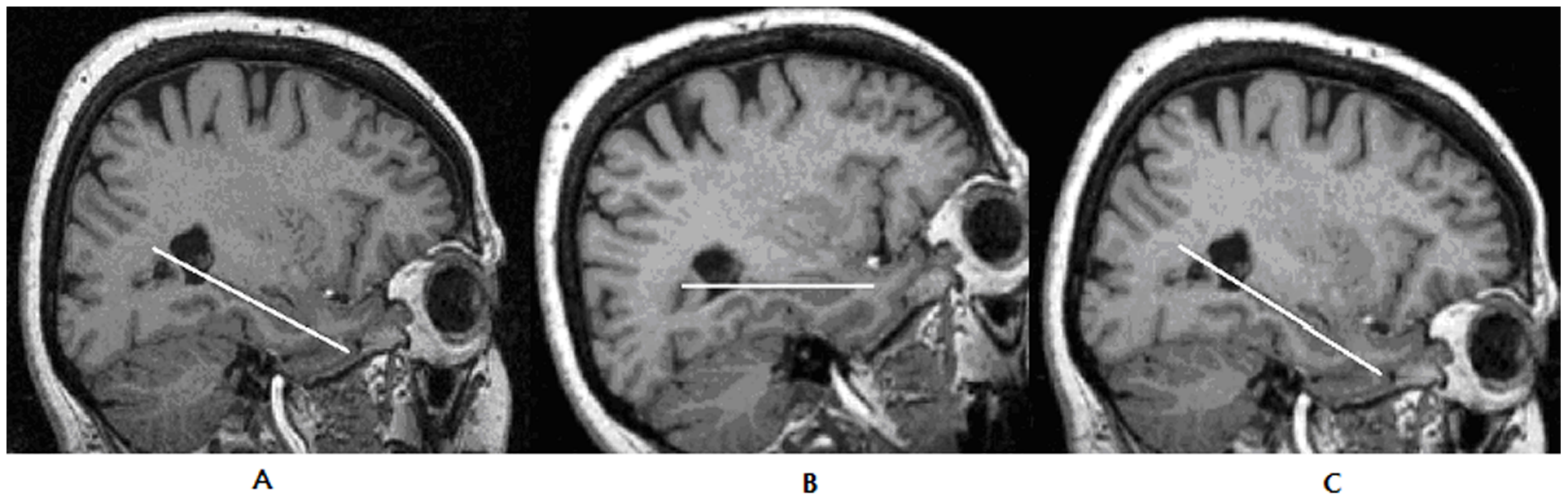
a,b,c Examples of the sagittal view of the right hippocampus on the MRI in one native and two reoriented axis. MRI sections were selected according to the best visibility of the of the hippocampus on the sagittal sections (its long or dorsoventral axis), a) position of the hippocampus on the “native” MRI scan, b) reorientation of the “native” MRI scan into the hipo-axis axis (where long axis of the hippocampus is parallel to the horizontal axis), c) reorientation of the “native” MRI scan into the CA-CP. White lines represent long axis of the hippocampus.
